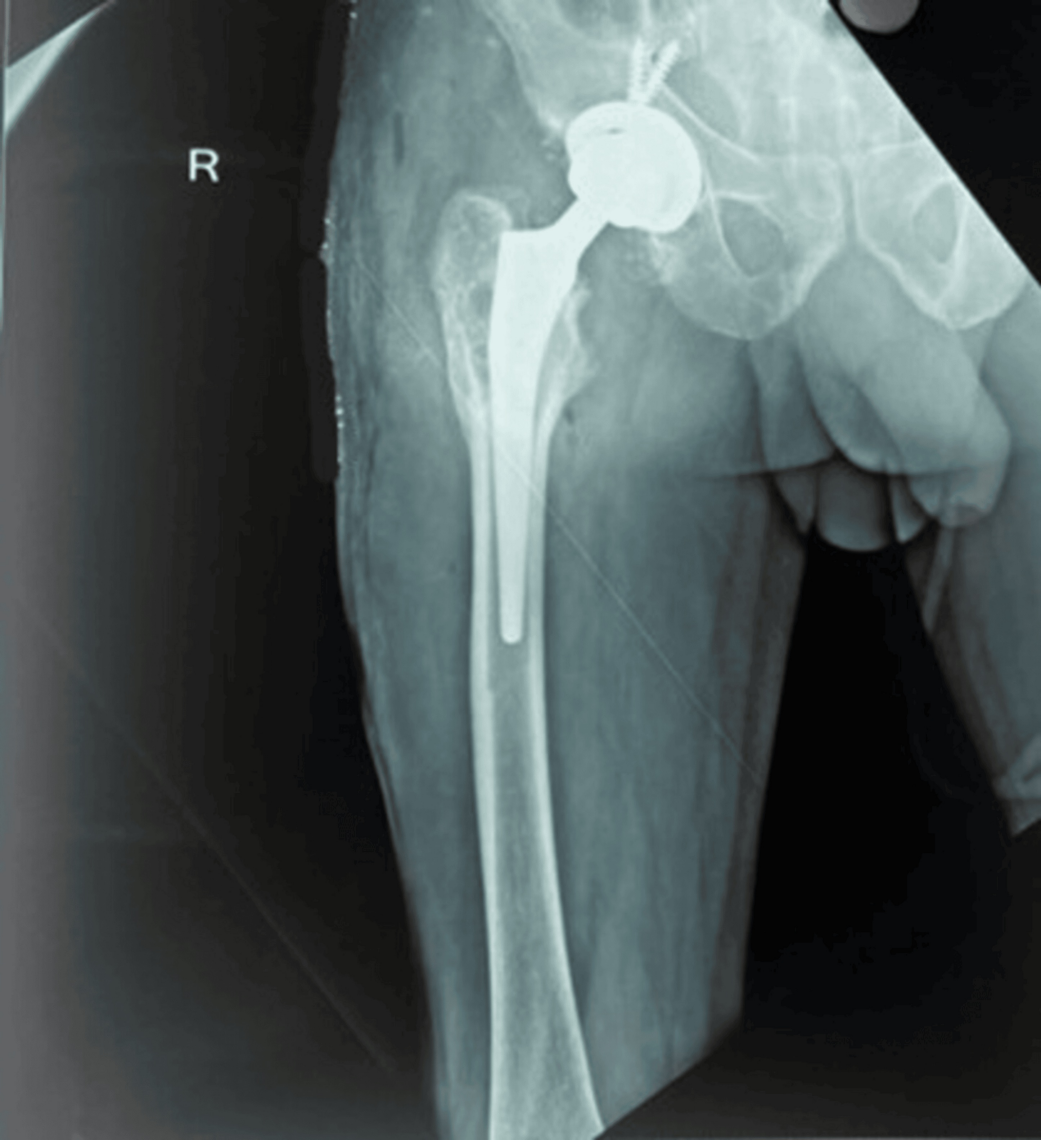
Case 2: Post-operative X-ray showing uncemented THR of the right hip. THR: total hip replacement
